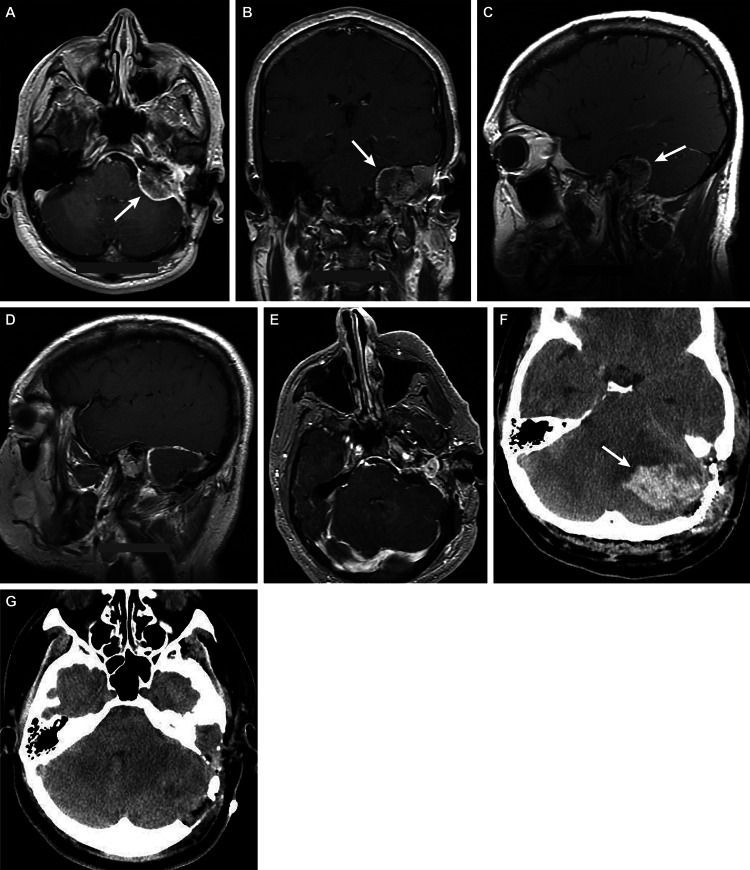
Case 3 – a 43-year-old man with a history of a recurring left metastatic glomus jugulare tumor (A) Axial CT and (B) coronal and (C) sagittal T1-weighted contrast-enhanced MRI indicated a left glomus jugulare tumor (arrows) measuring 3.3 × 4.7 × 4.1 cm in the anteroposterior, transverse, and craniocaudal dimensions. The tumor involved the petrous apex, with increased mass effect on the left cerebellopontine (CP) angle but no evidence of midline shift. The mass extended into the left middle ear, external auditory canal, and jugular foramen. Postoperative (D) sagittal and (E) axial T1-weighted contrast-enhanced MRI showed expected postoperative changes related to a left retrosigmoid craniotomy for debulking of the left CP angle mass. There was decreased mass effect, and a residual 1.6 × 1.7-cm nodular enhancement could be visualized in the resection cavity. (F and G) Axial CT on postoperative day three showed postoperative changes and revealed a new 4.6 × 2.7-cm left cerebellar hematoma (F, arrow), resulting in compression of the fourth ventricle and foramen magnum, brainstem (right midline) shift, and mild supratentorial herniation MRI: magnetic resonance imaging; CT: computed tomography Used with permission from Barrow Neurological Institute, Phoenix, Arizona
